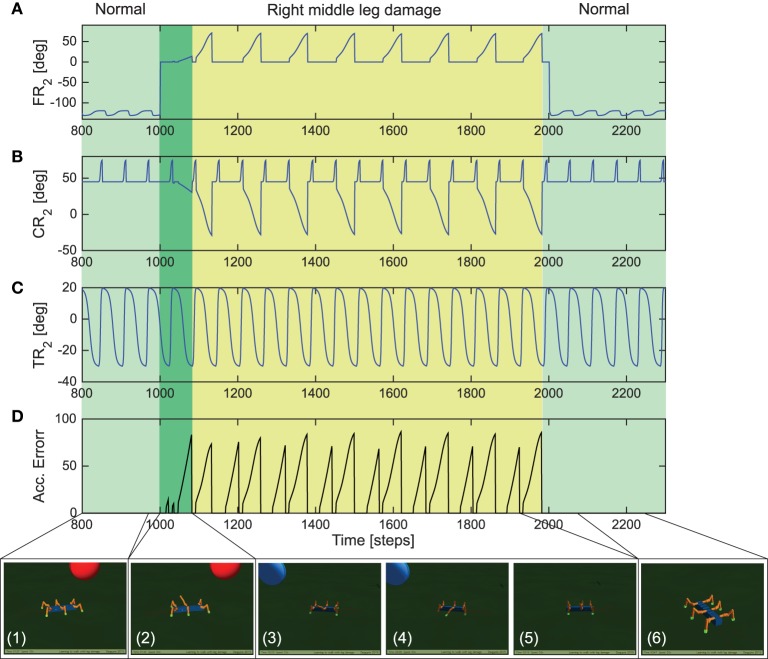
Real-time data for adaptive locomotion to overcome leg damage. (A) The FT-i joint angles of the right middle leg *R*_2_. (B) The CT-i joint angles of the right middle leg *R*_2_. (C) The TC-i joint angles of the right middle leg. (D) Accumulated error signal at the end of each stand phase. It is reset to zero at every swing phase. Below pictures show the locomotion of AMOSII during the experiment (temporal spacing of the panels are not exact). Please see the Supplementary Video 6 for closer look at the exact adaptive behavior.
